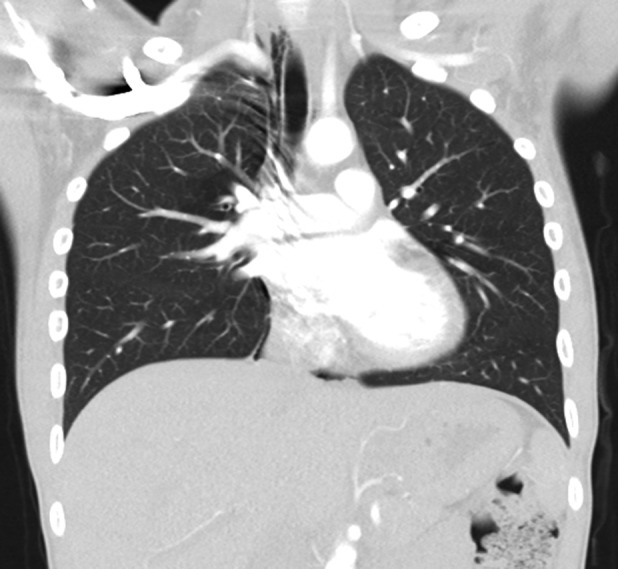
Coronal computed tomography scan (Toshiba Aquilion 64, acquisition parameters: 120 kV, 20 mA) shows a significant mediastinal emphysema more pronounced on the right side.
